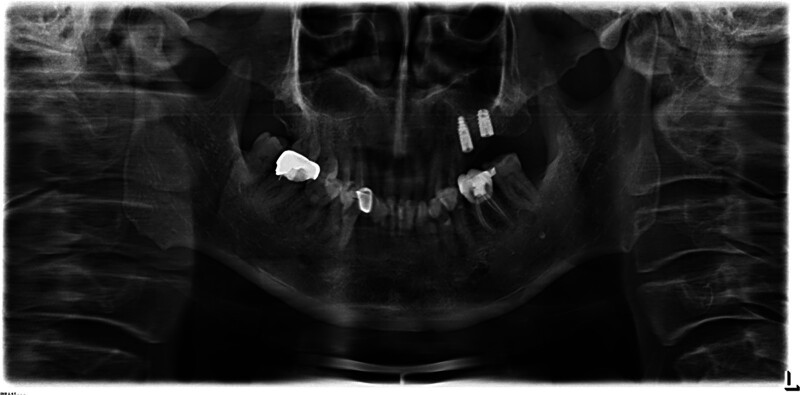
The oral panoramic radiograph shows the postoperative appearance of implant placement after the loss of the first and second maxillary molars on the left side, and a slight decrease in the density of the alveolar bone around the root apex of the distal root of the right mandibular first molar.
